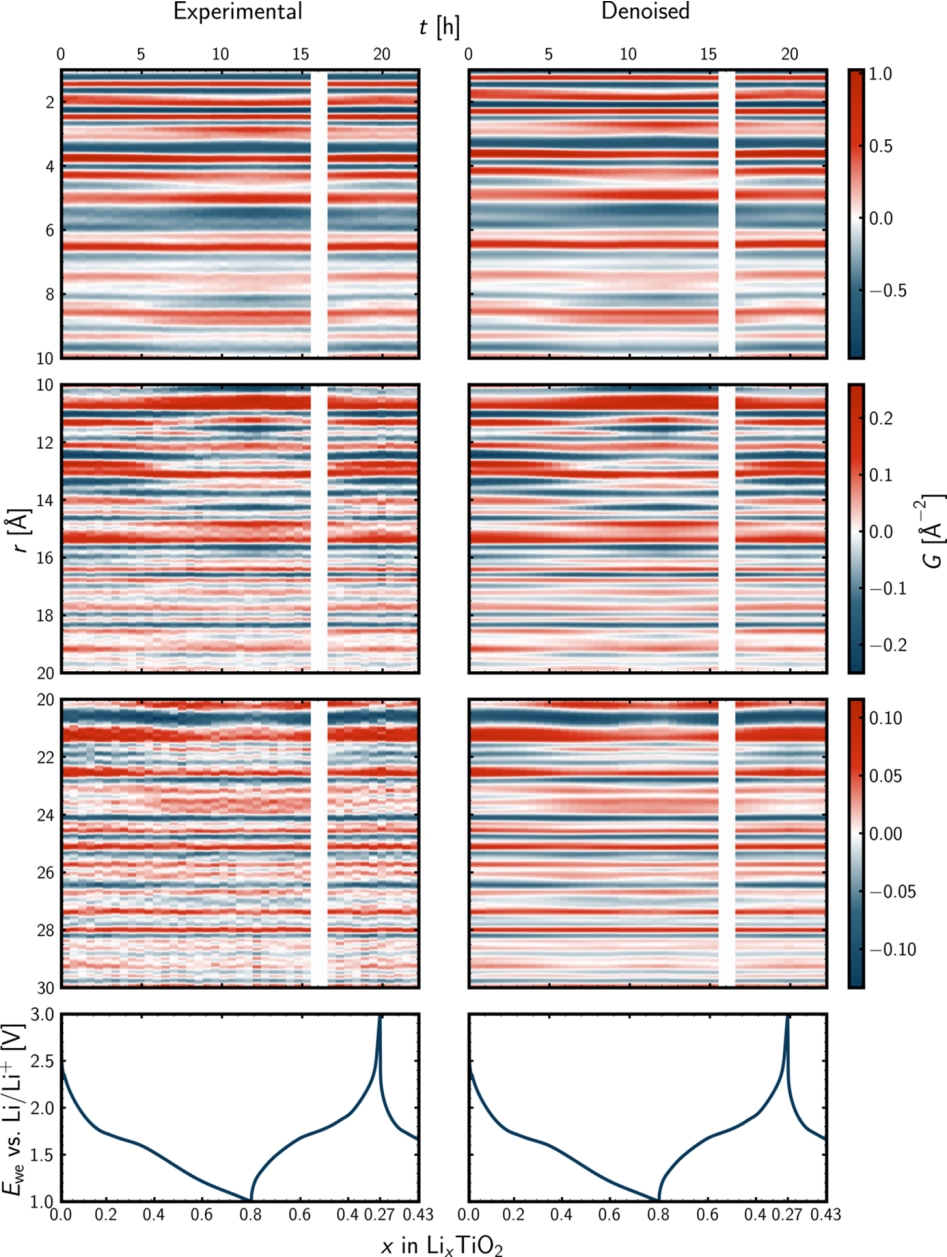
Experimental (left) and denoised (PCA-reconstructed, right) *operando* PDF data together with galvanostatic cycling. The reduced atomic PDF, *G*, as a function of the interatomic distance, *r*, and time, *t*, during the *operando* experiment. Note the different scales for the colour bars of the different *r* ranges. The white columns represent the absence of synchrotron X-rays during the *operando* experiment. For the voltage profile, the electrochemical potential of the working electrode, *E*_we_ versus Li/Li+, is shown as a function of the state of charge, *x*, in Li_*x*_TiO_2_.
